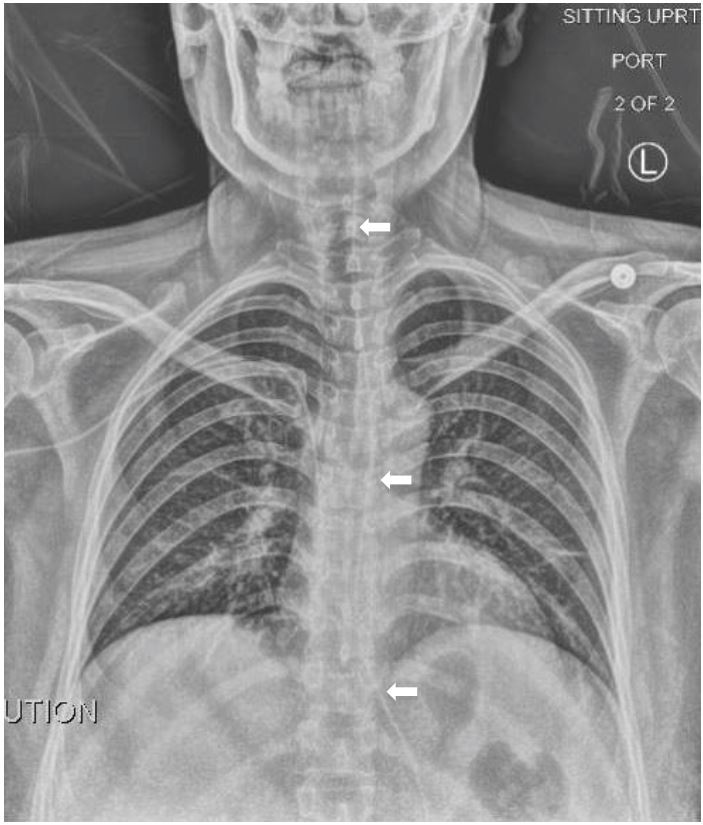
Upright chest radiograph with visible gastrojejunostomy tube superiorly displaced up the esophagus.
